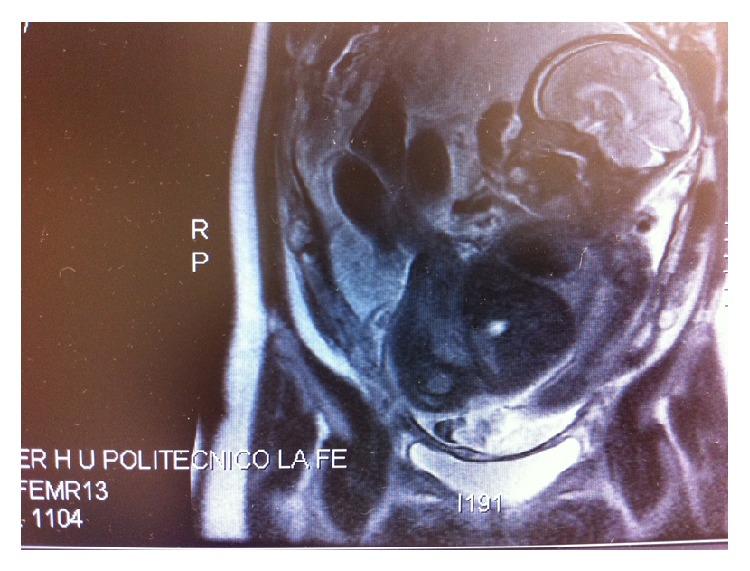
Magnetic resonance imaging of the fetal cyst.
